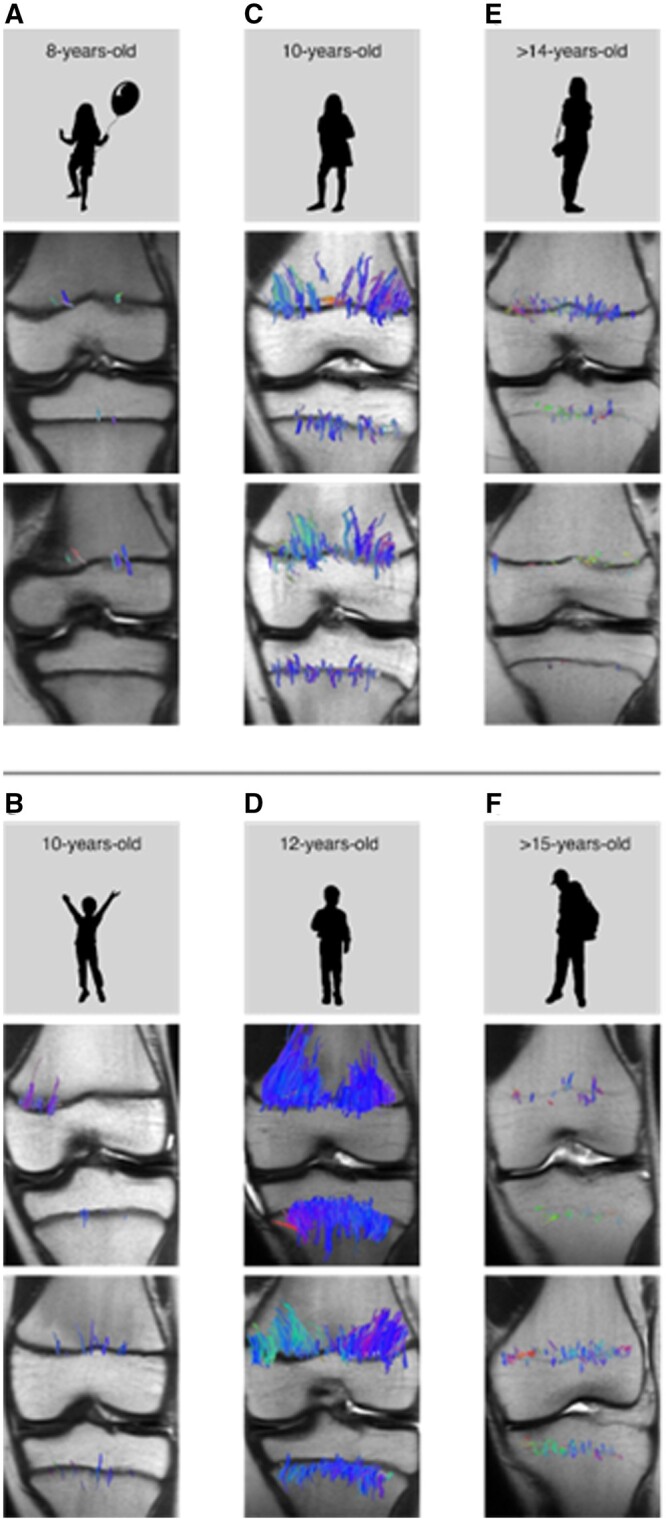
Tractography superimposed on T1-weighted images in children transitioning into puberty: 8-year-old females (A) and 10-year-old males (B). Femoral and tibial fibre tracts are scant, short, and parallel to the long axis of the bone in both females and males. In children experiencing peak growth, the physeal tractography pattern increases in density and thickness because these children are undergoing rapid bone growth. Rapid cell proliferation and hypertrophy results in both increased chondrocyte column height and increased stature. In this phase the columns are longer, more densely packed, and organized in parallel, characteristics that are reflected in the tractography pattern (C, D). After the growth spurt the tracts becomes less dense, shorter and more asymmetrical (E, F).
